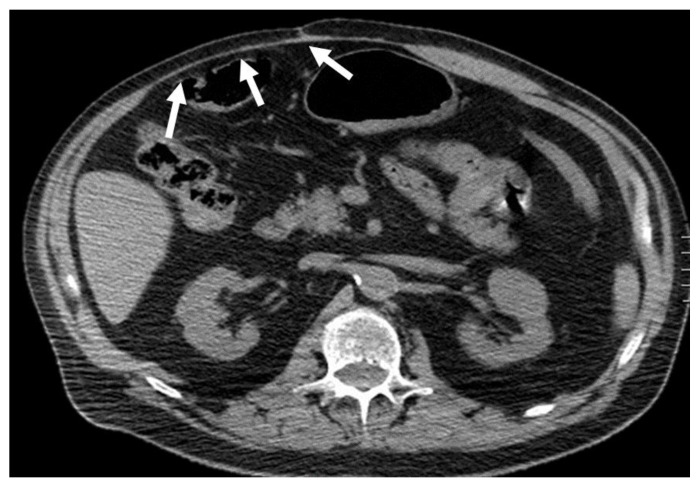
Axial, not-enhanced CT scan shows the congenital absence of the right rectus muscle (arrows).
